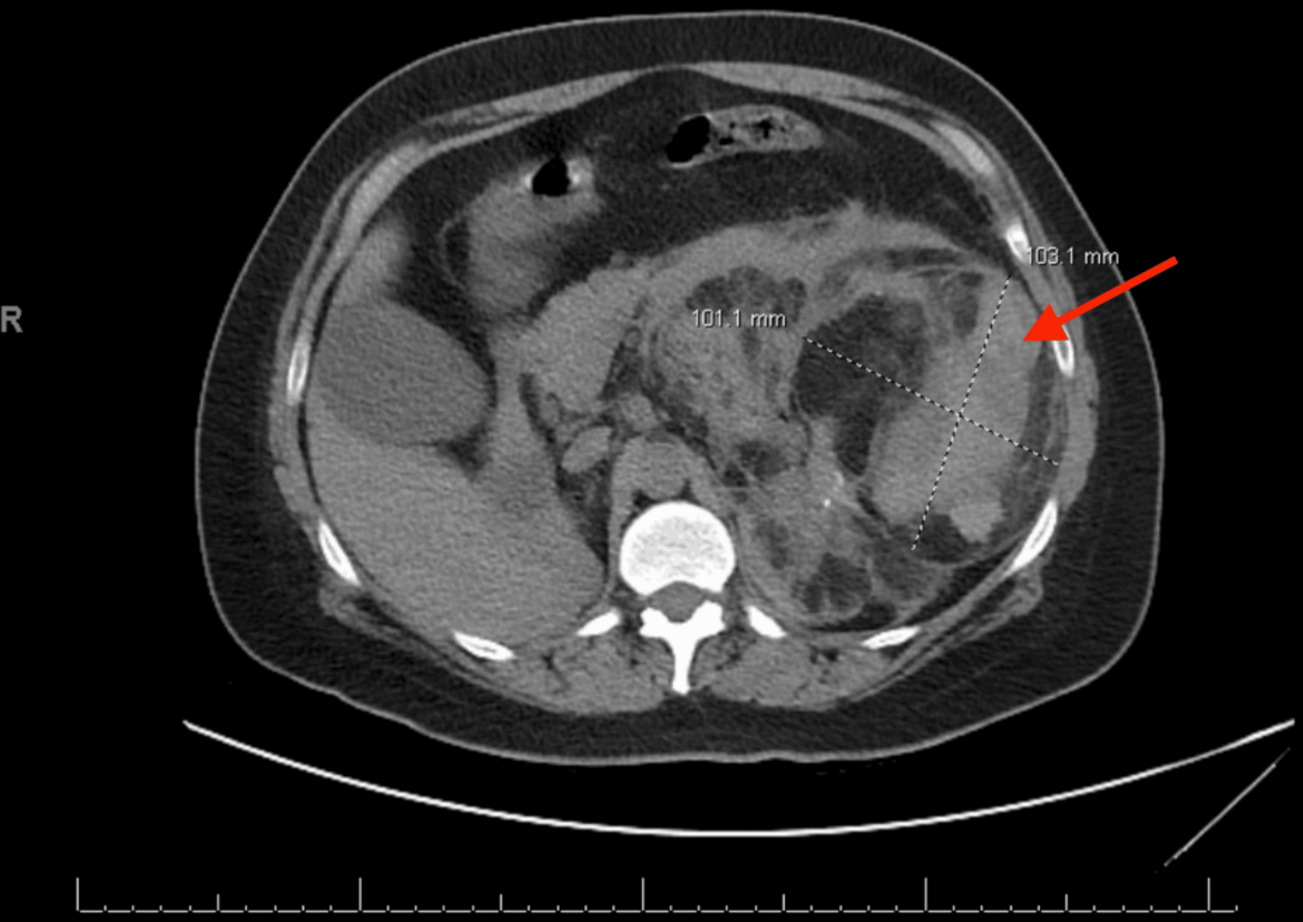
CT angiogram of the abdomen and pelvis: large left angiomyolipoma essentially replacing left renal parenchyma, measuring 12.8 x 12.8 x 21 cm with left perinephric hematoma in the axial plane
